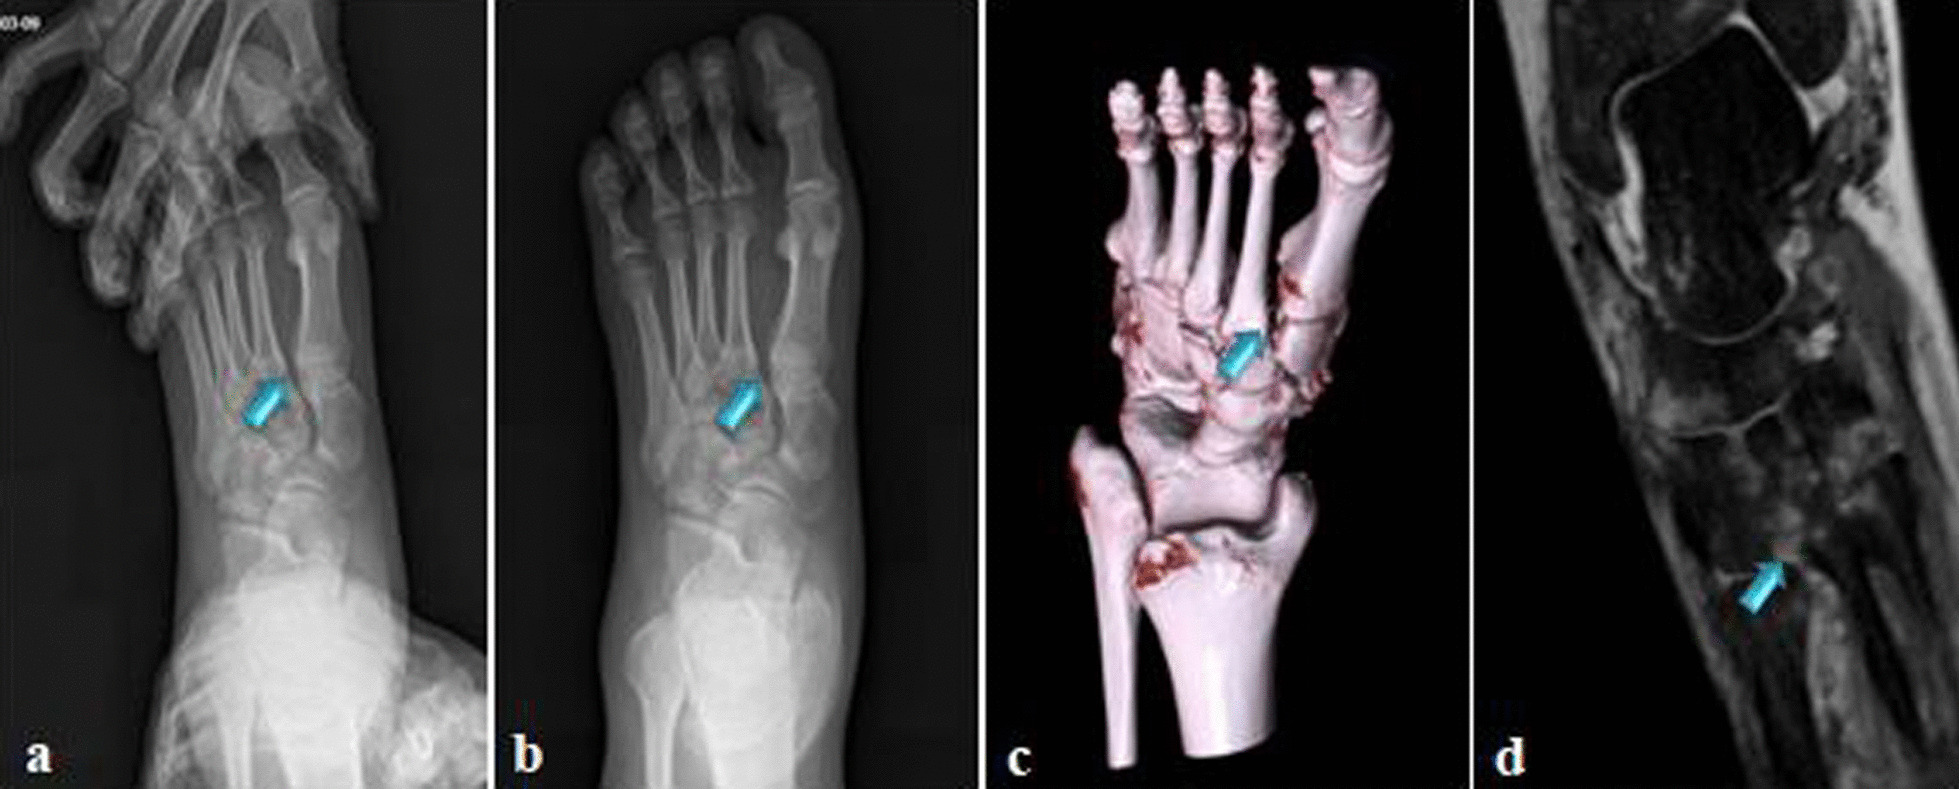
a–d Preoperative stress view, anteroposterior (AP) foot radiograph, weight-bearing CT and MRI of 27-year-old male patient with purely Lisfranc ligament injuries (blue arrows showing injury)
